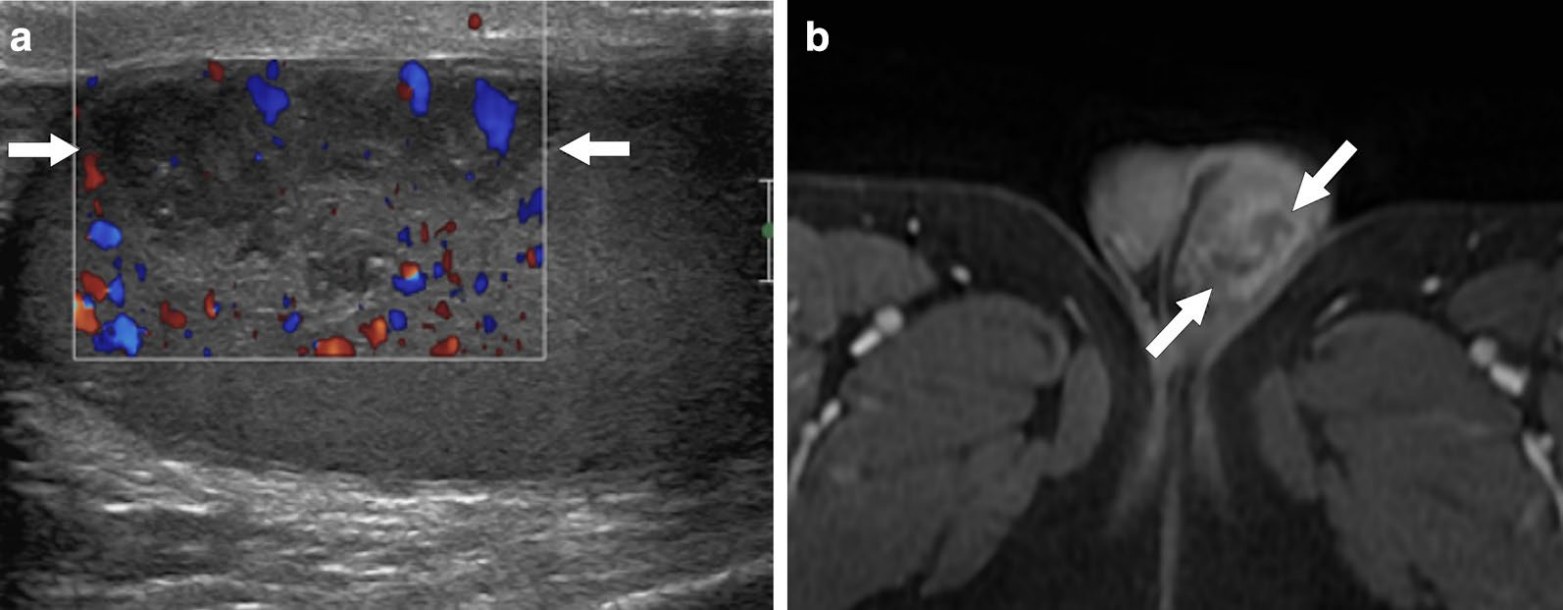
Testicular abscess. A 30-year-old healthy male with testicular pain and palpable scrotal mass. Clinical history and physical exam, apart from palpable left testicular mass, were unremarkable. a Color Doppler ultrasound image demonstrates a complex cystic mass with increased peripheral and mild central vascularity (arrows). b Postcontrast fat suppressed T1W axial plane image shows predominantly peripherally enhancing left testicular mass (arrows). Polymicrobial abscess was pathologically confirmed after orchiectomy. No obvious underlying risk factor could be discerned for testicular abscess
